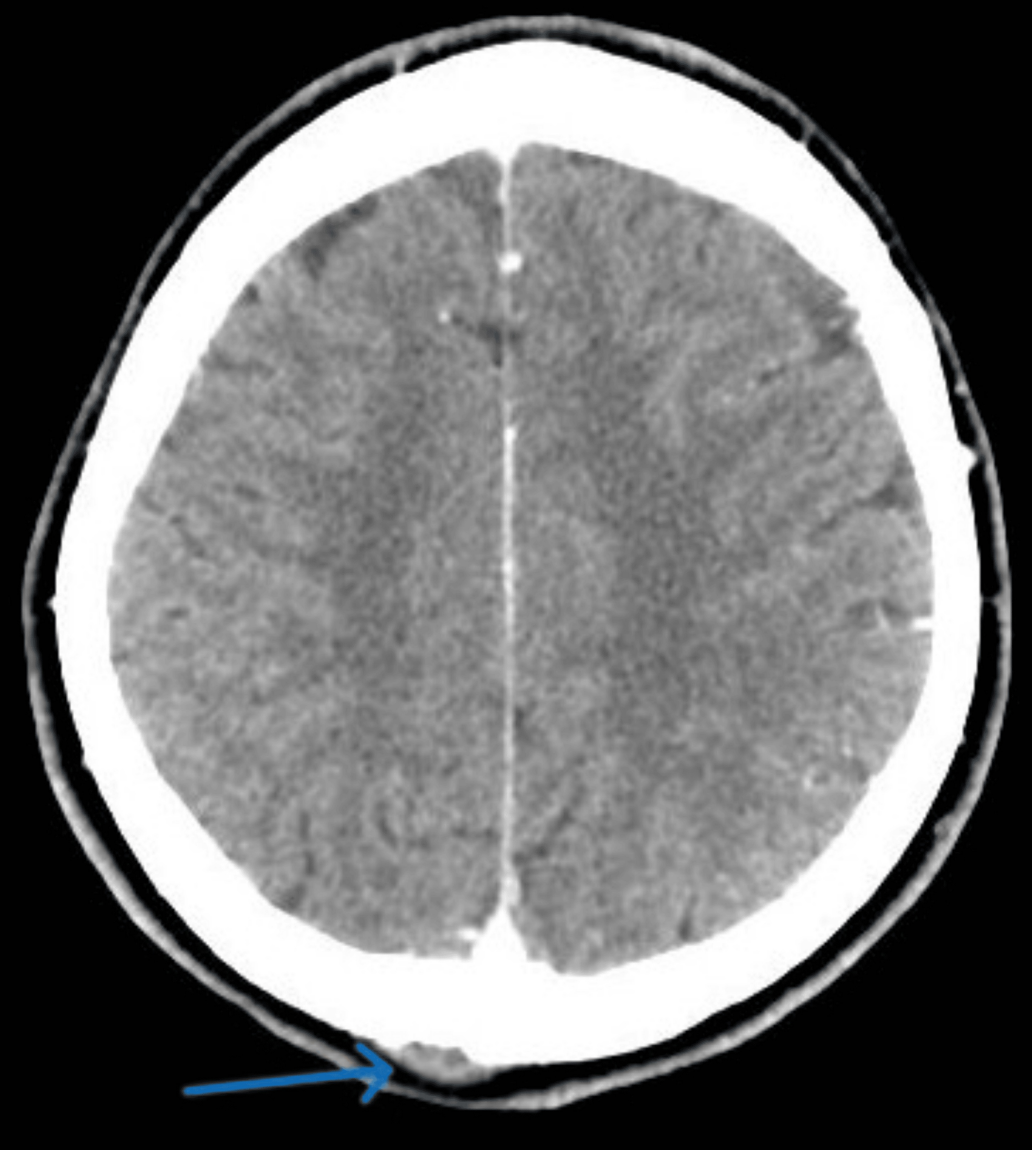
An axial CT scan of the brain with contrast demonstrates a lytic lesion in the occipital bone with a small extracranial soft tissue component.
